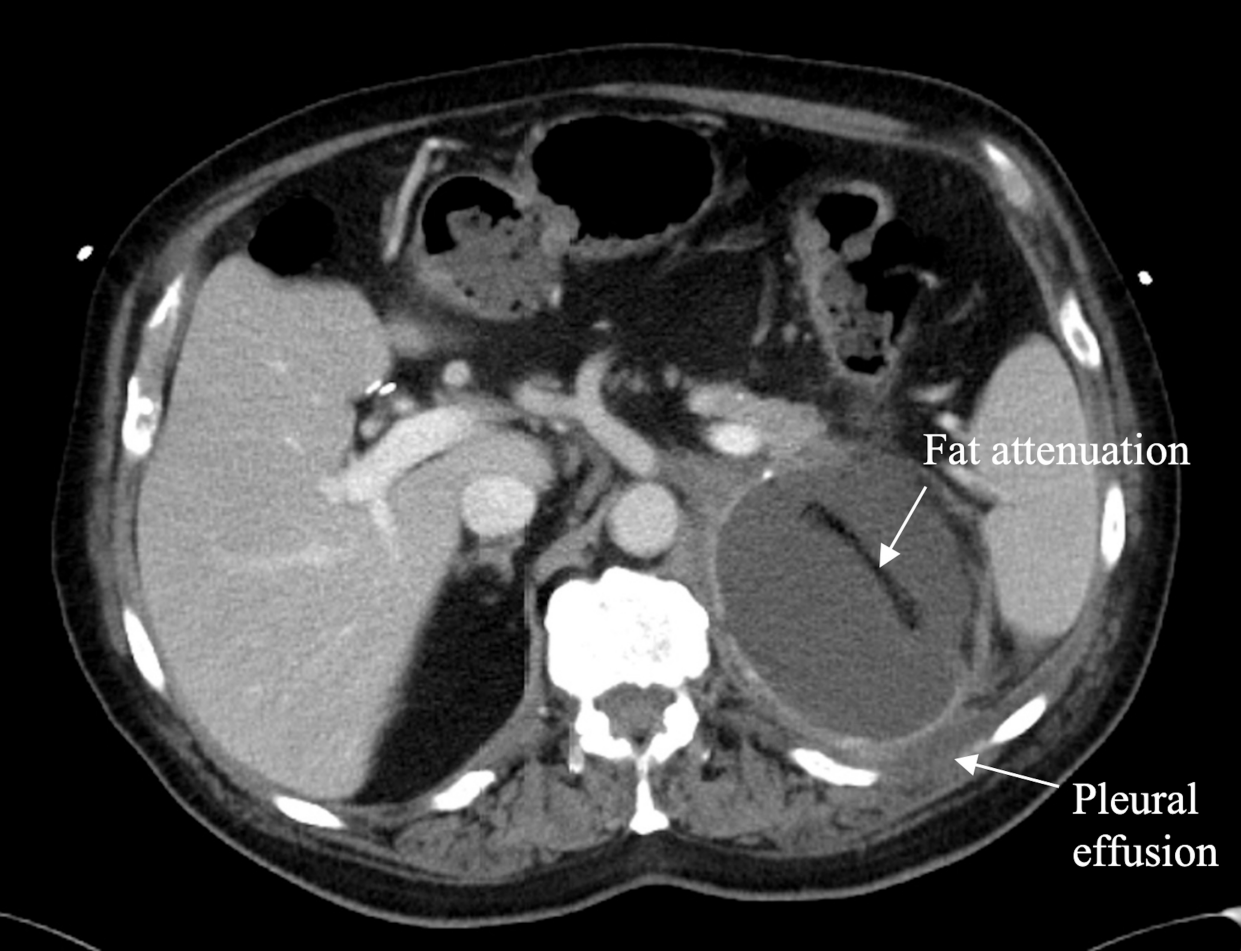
Axial contrast enhanced CT through the upper abdomen in June 2023 shows complete replacement of the left adrenal gland by a well circumscribed fluid attenuation mass with smooth margins measuring 9.9 x 7.9 x 7.8 cm. A linear area of fat attenuation is seen within the mass along the path of a previously placed pigtail catheter. There is a small left pleural effusion. The right adrenal mass has resolved, and the right adrenal gland appears normal.
